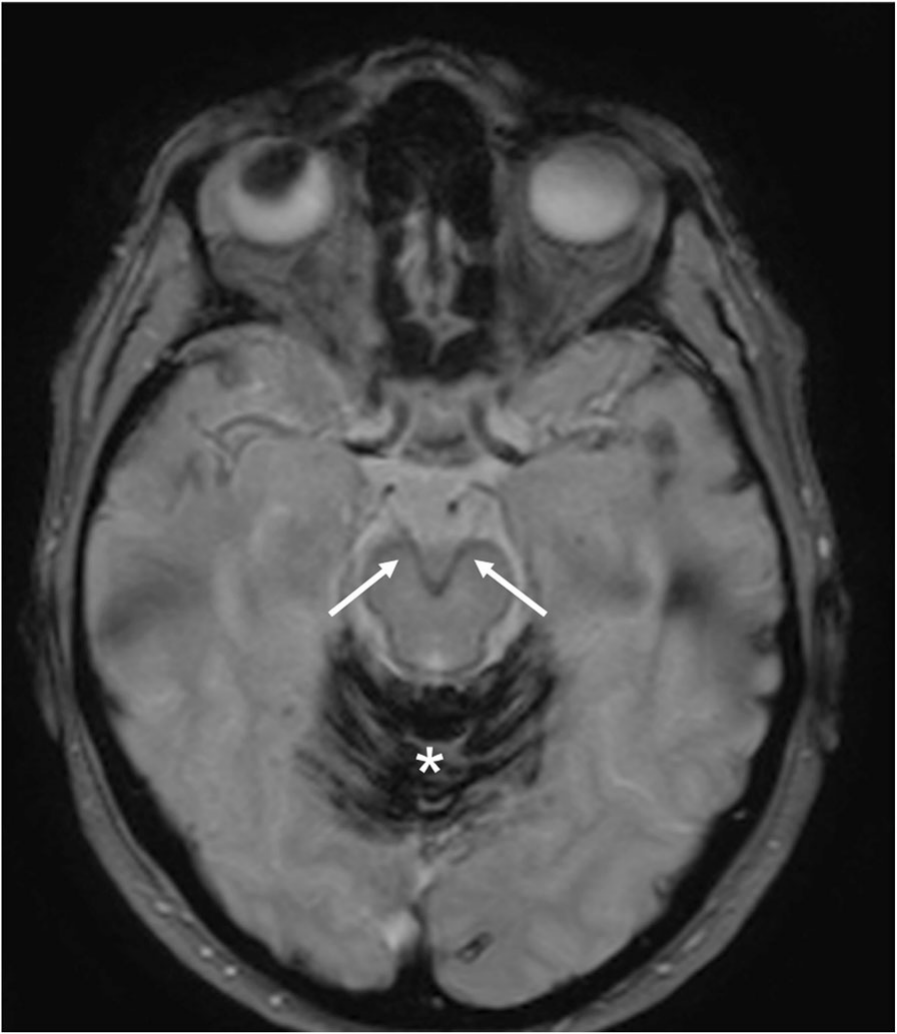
Superficial siderosis. MRI T2*-weighted axial image demonstrates hemosiderin deposition along the course of oculomotor nerves (arrows). Massive hemosiderin deposition is also visible on the cerebellar folia (asterisk)
